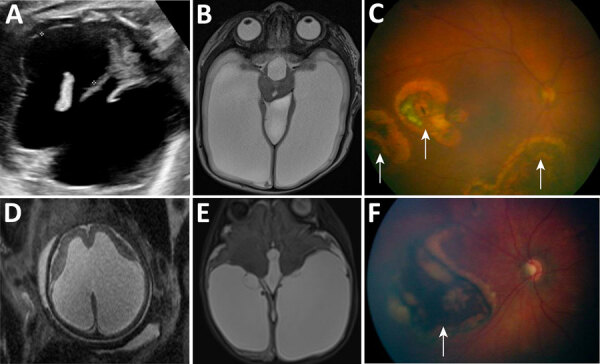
Imaging findings for ventriculomegaly and chorioretinitis associated with congenital lymphocytic choriomeningitis virus infection, Philadelphia, Pennsylvania, USA. A–C) Case 1; D–F) case 2. A) Routine ultrasonography at 36 weeks’ gestation, demonstrating severe cerebral ventriculomegaly in the fetus (black space between markers on the anatomic right and the unmarked black space on the anatomic left). B) Postnatal (38 weeks 4 days’ gestation) brain magnetic resonance imaging of newborn confirmed severe cerebral ventriculomegaly (large, white, fluid-filled spaces bilaterally and in the center of the image). C) Fundoscopic examination of newborn, revealing multiple areas of chorioretinitis (arrows). D) Routine ultrasonography at 36 weeks’ gestation, demonstrating severe cerebral ventriculomegaly in the fetus. E) Postnatal (38 weeks 5 days’ gestation) brain magnetic resonance imaging of newborn confirmed severe cerebral ventriculomegaly. F) Fundoscopic examination of newborn, revealing a large area of chorioretinitis (arrow).
